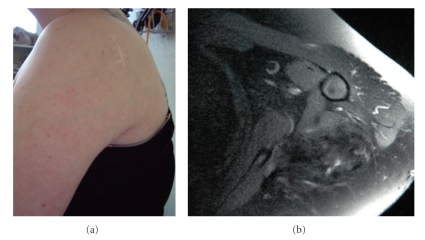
Clinical response after Imatinib therapy: Recovery of the whole curving contour of the shoulder, with decrease of collateral circulation (a). MRI findings: T1 gadolinium sequences confirming the tumor very good partial response with disappearance of tumor-related hypersignal (b).
